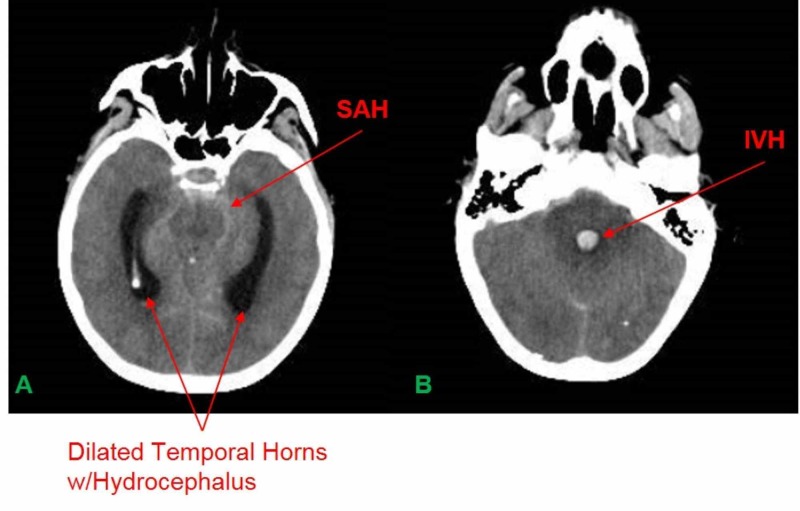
A. CTH revealing SAH and dilated temporal horns w/hydrocephalus. B. CTH revealing IVH in the 4th ventricle CTH = CT Scan of Head; SAH = Subarachnoid Hemorrhage; IVH = Intraventricular hemorrhage
